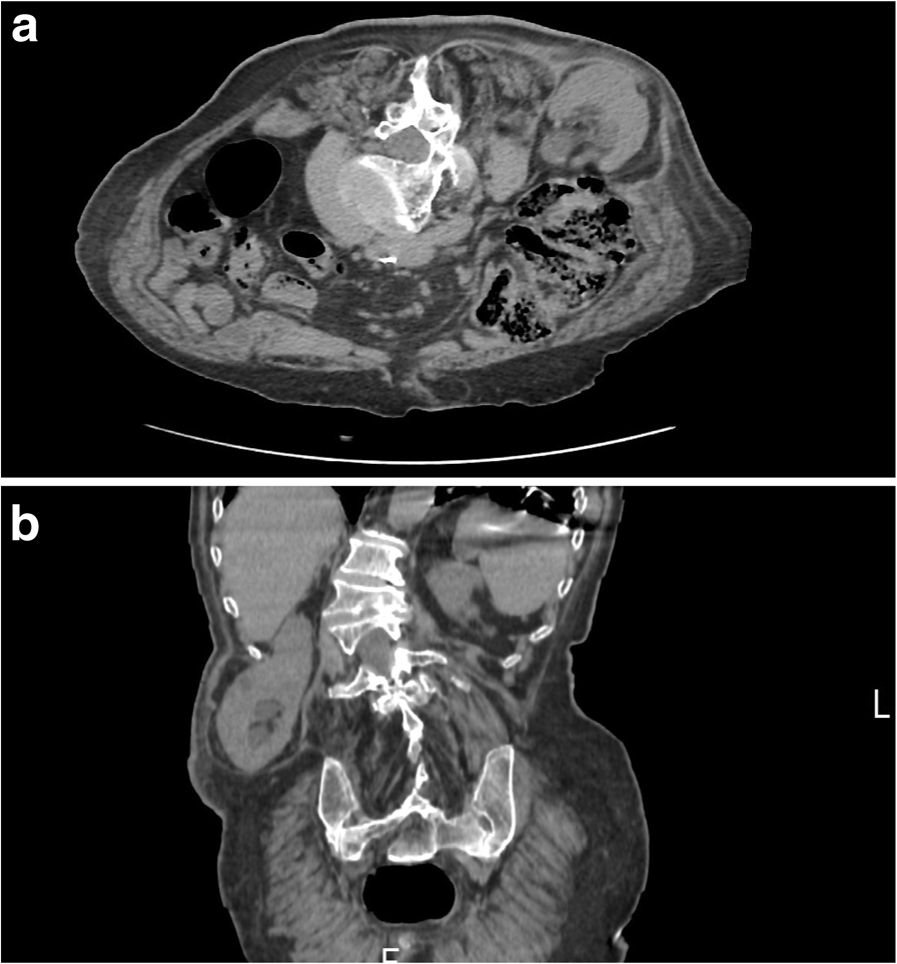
Computed tomographic images without contrast media. a Axial plane. b Sagittal plane. Images show a right inferior lumbar hernia (Petit hernia) through the inferior lumbar triangle between quadratus lumborum muscle, external oblique muscle, and the left iliac muscle, containing adipose tissue and the right kidney
